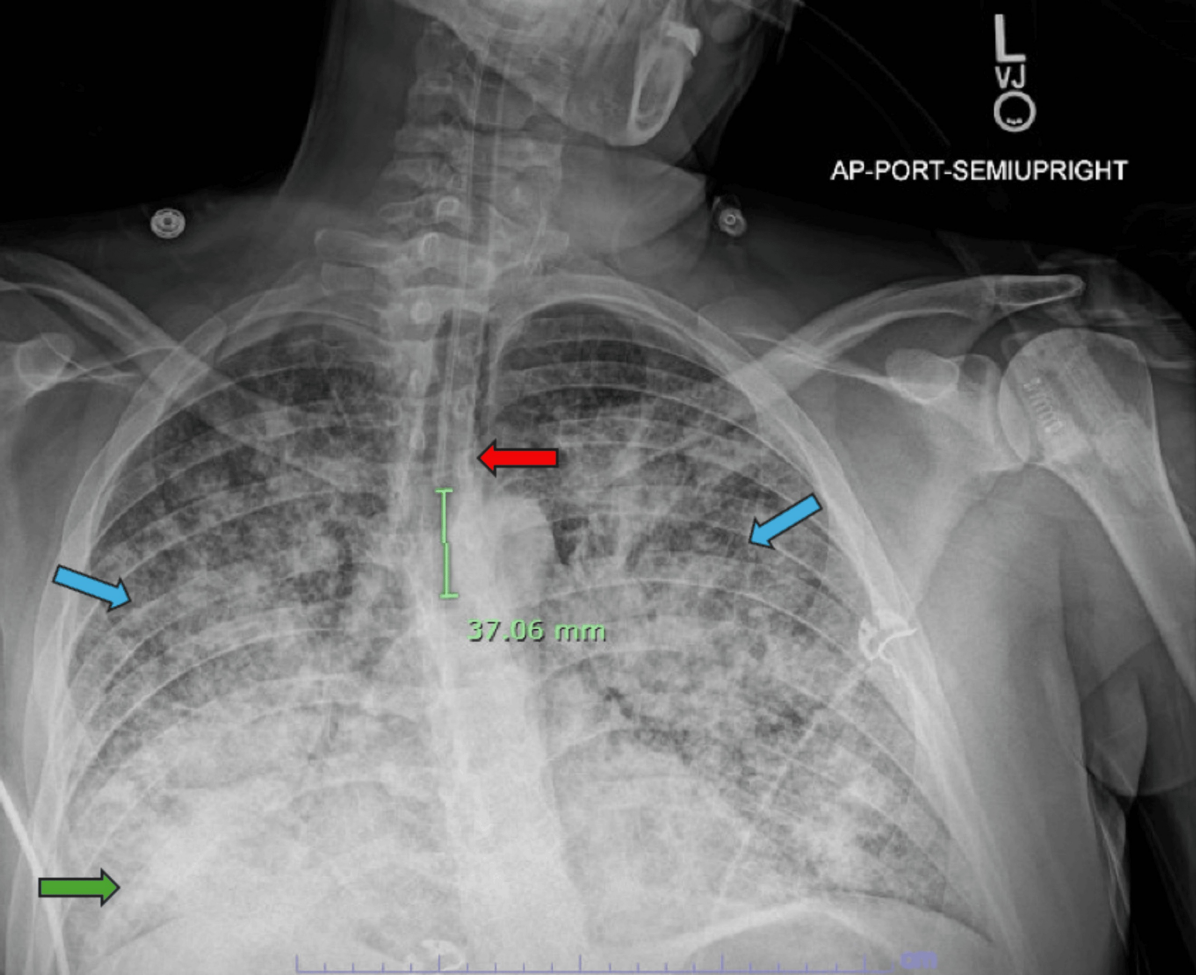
Repeat CXR showing worsening right lower lobe consolidation (green arrow) and bilateral reticulonodular opacities (blue arrows) with endotracheal tube (red arrow) terminating 3.7 cm above the carina CXR: Chest X-ray
